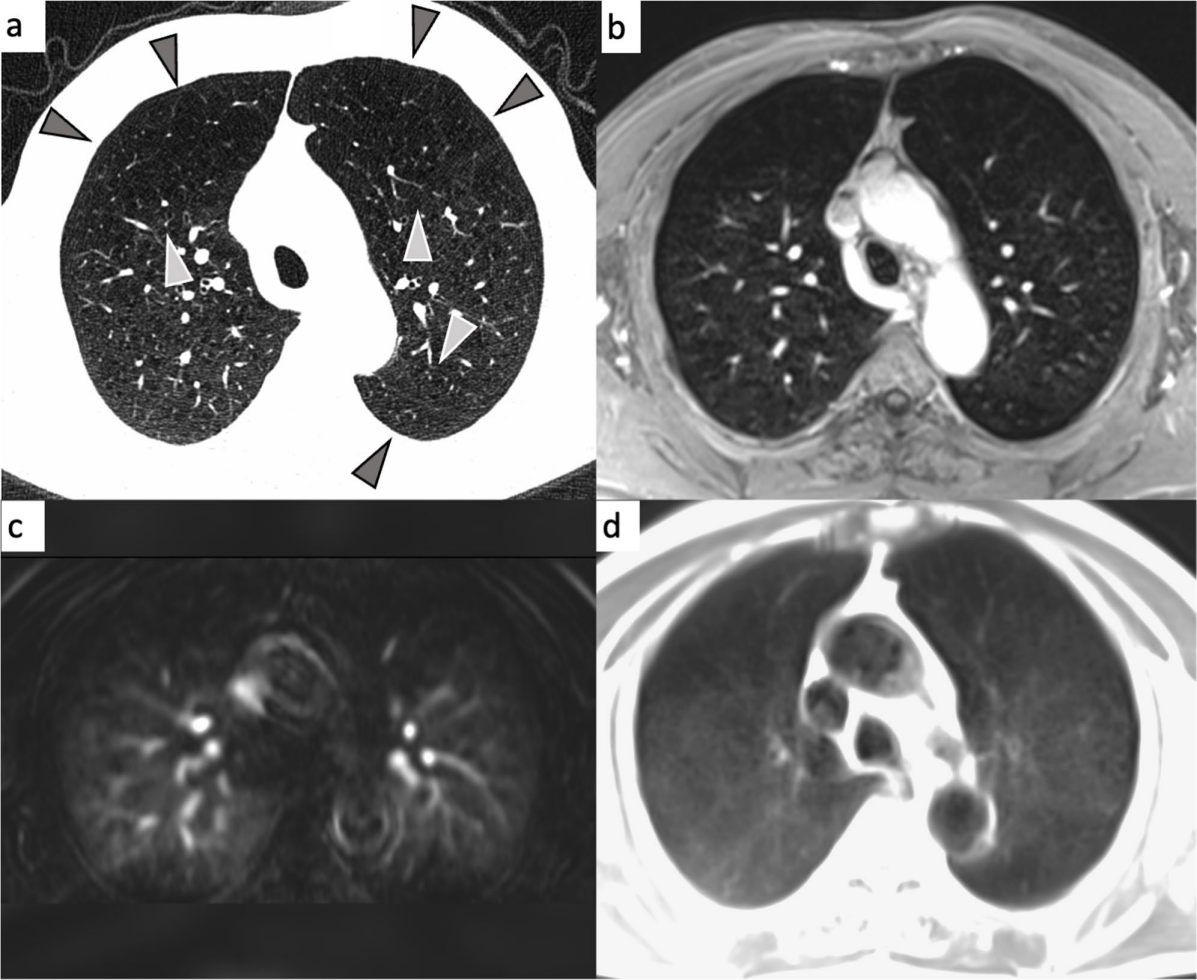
Emphysema-type COPD in a 69-year-old male participant (classified as emphysema predominant COPD phenotype). Arrowheads on the CT image (a) indicate severe emphysema in both anterior upper lung lobes and in the tip of the left lower lung lobe. On the corresponding MRI, the affected parts of the lung appear as lung parenchyma defects on the contrast-enhanced transverse 3D gradient echo image (b), as perfusion deficits in the DCE series (c) and as signal void in the transverse half Fourier fast spin echo image (d)
